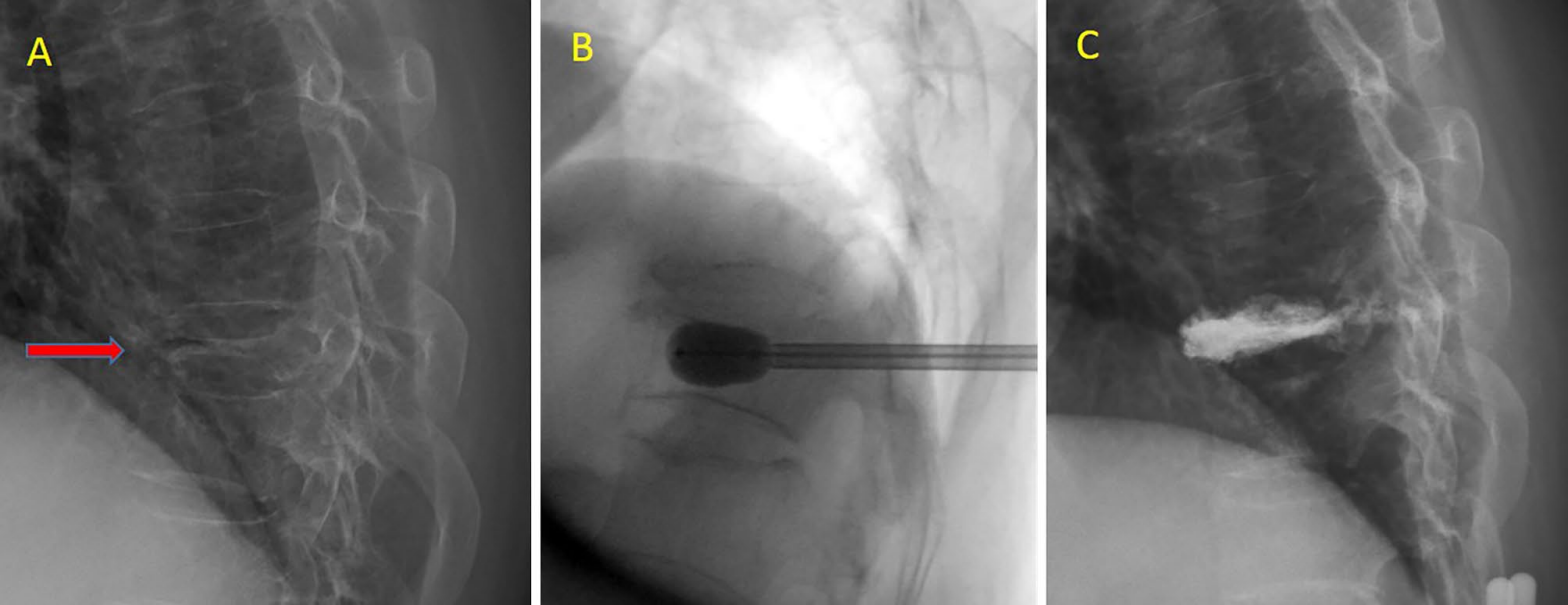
A case of T9 vertebral compression fracture treated with low-viscosity cement balloon kyphoplasty. (A) The arrow in the preoperative lateral radiograph points to the fracture level. (B) Intraoperative radiograph shows the balloon inflated in the fractured vertebrae. (C) Postoperative lateral radiograph demonstrates cement filled in the fractured vertebrae.
